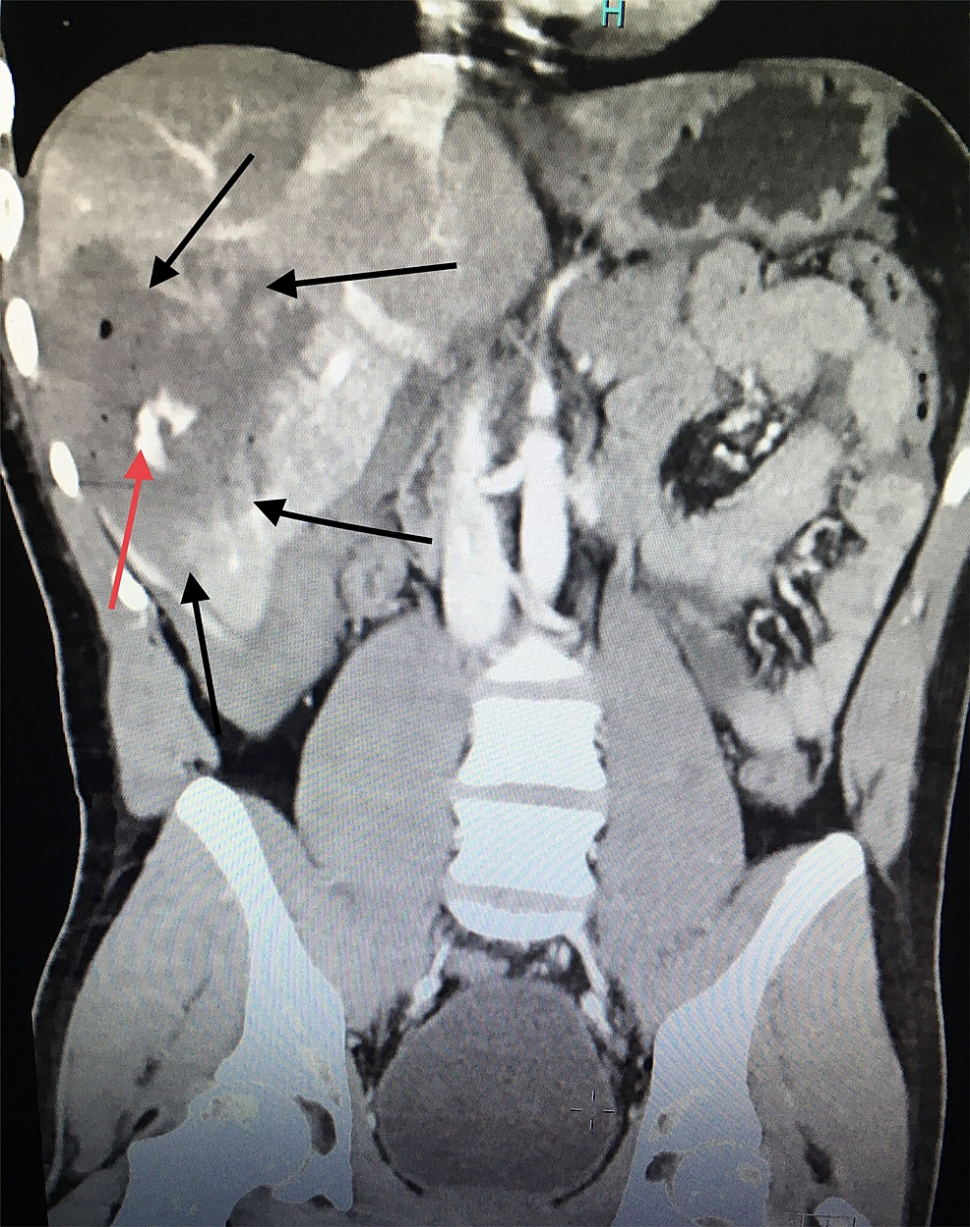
CT angiogram of the abdomen (coronal plane). Pseudoaneurysm: contrast outside the vessel depicted by the red arrow. Grade IV liver injury depicted by the black arrow.
